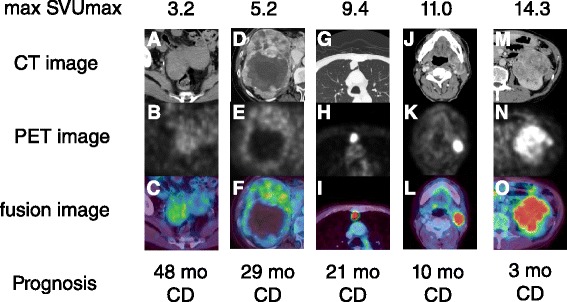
The features of FDG PET/CT and prognosis. (*a*, *b*, *c*) A case with ovarian recurrence and a max SUVmax of 3.2. (*d*, *e*, *f*) A case with a primary tumor and a max SUVmax of 5.2. (*g*, *h*, *i*) A case with lung metastasis and a max SUVmax of 9.4. (*j*, *k*, *l*) A case with submandibular lymph node metastasis and a max SUVmax of 11.0. (*m*, *n*, *o*) A case with a primary tumor and a max SUVmax of 14.3. *a d g j m*: CT imaging. *b e h k n*: PET images. *c f i l o*: fusion images. mo=month. CD=cancer death
